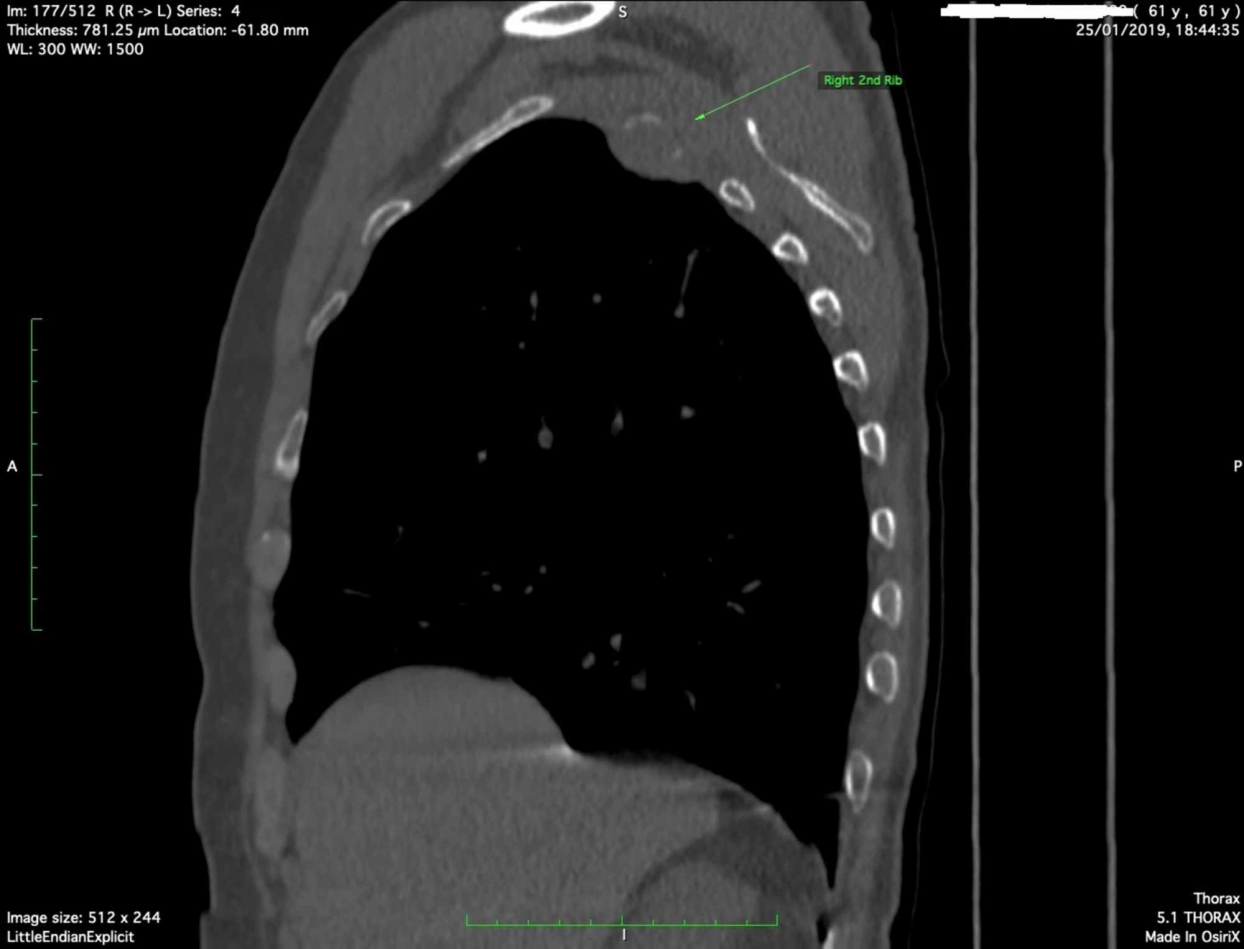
Axial view of CT thorax showing an osteolytic area in the second rib with a surrounding mass
